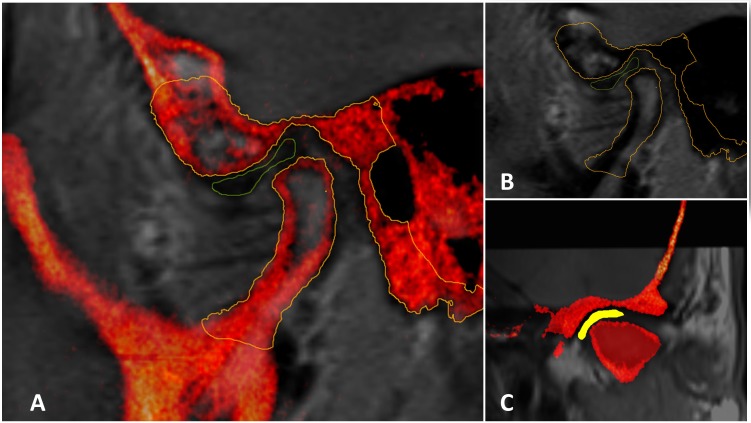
Tissues segmentation. A: Oblique sagittal PD-weighted MRI(gray)-CBCT(red) registered image showing outlined/segmented articular disc and condylar head and TMJ structure of the temporal bone. B: Oblique sagittal PD-weighted MRI only showing the outlined/segmented osseous structures from the co-registered CBCT. C: Oblique coronal PD-weighted MRI-CBCT registered image showing the same outlined/segmented structures.
